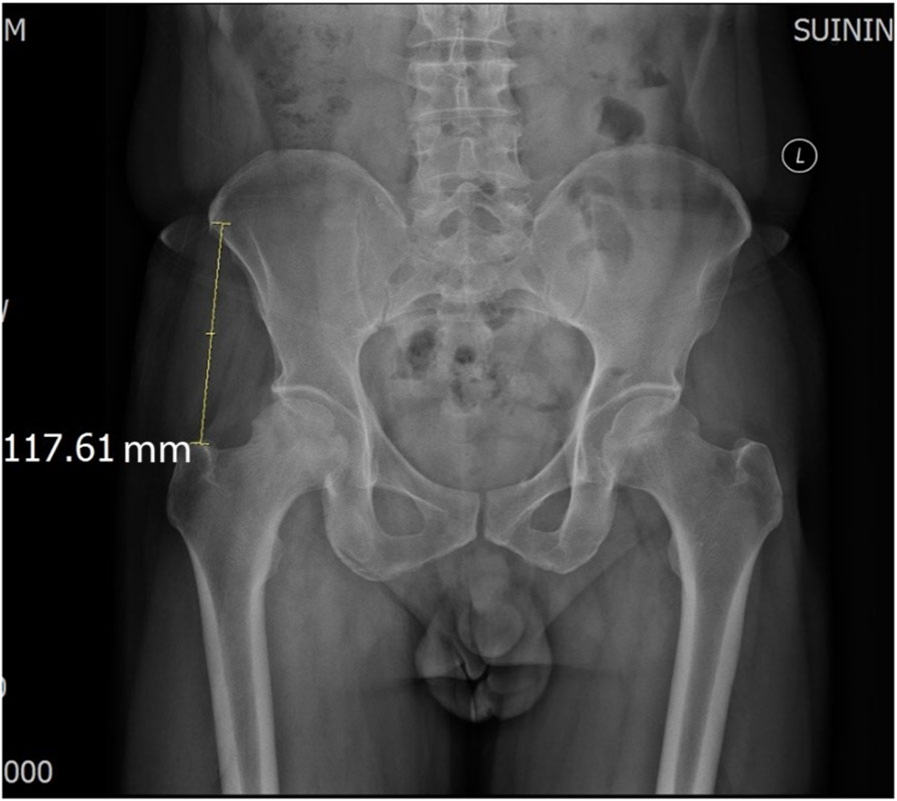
ASIS-GTD: the distance between the anterior superior iliac spine and greater trochanter, which was measured on standard supine pelvic X-rays
